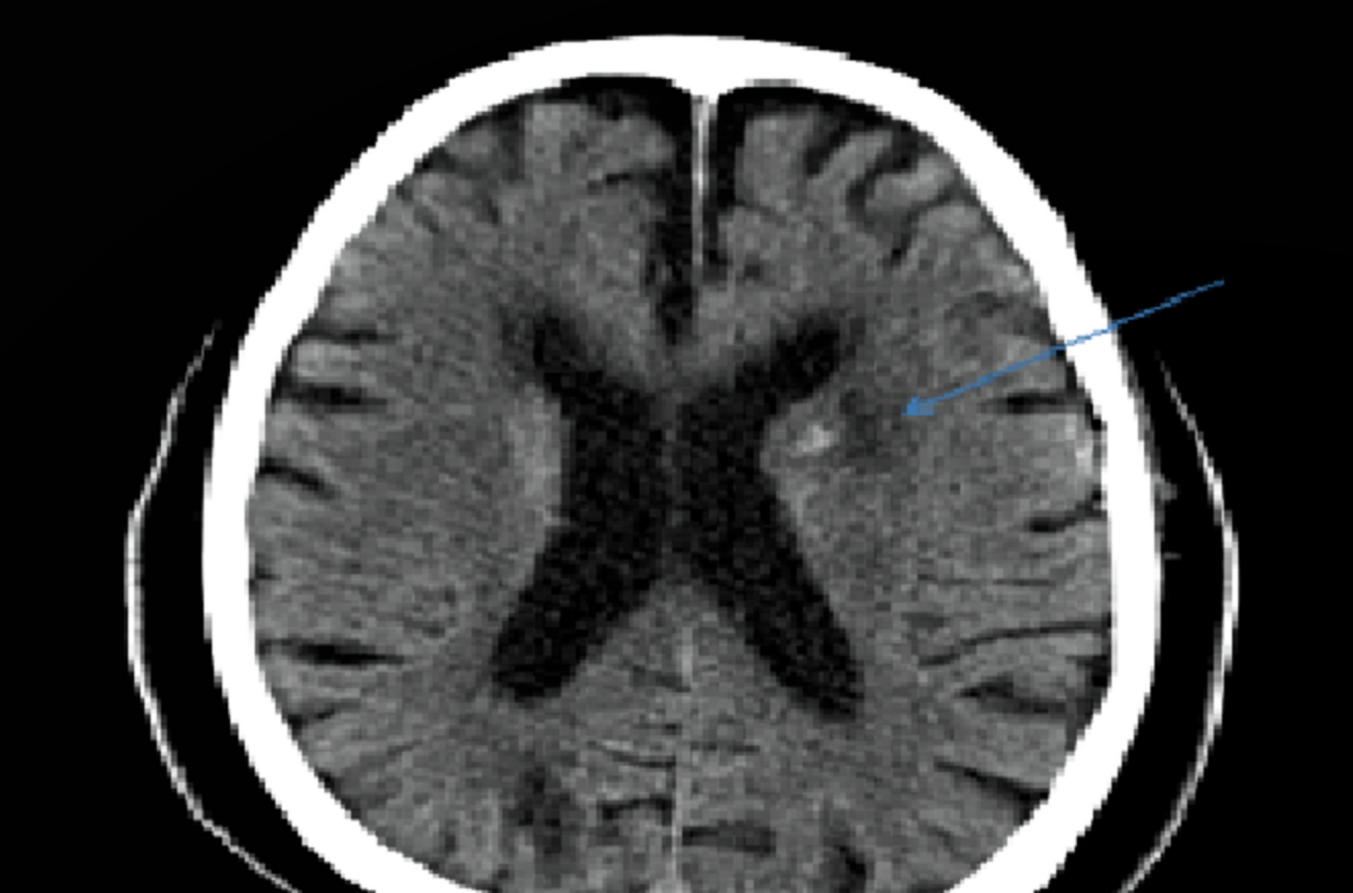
Computed tomography (CT) head without contrast showing acute ischemic infarction in the left caudate/putamen area.
